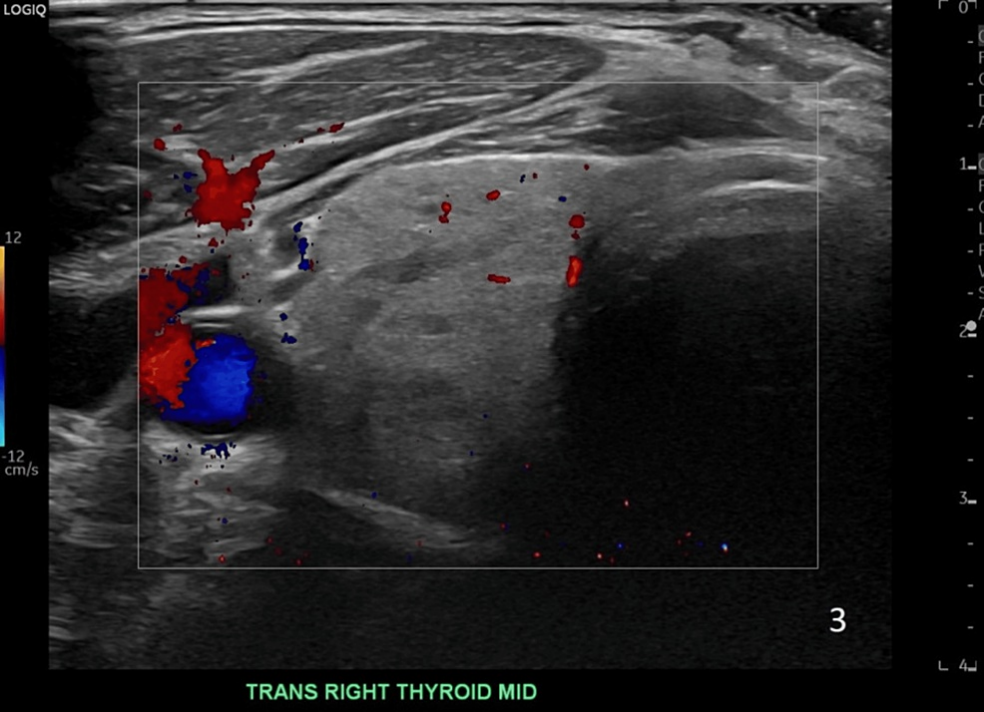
Image of the right lobe thyroid ultrasound
